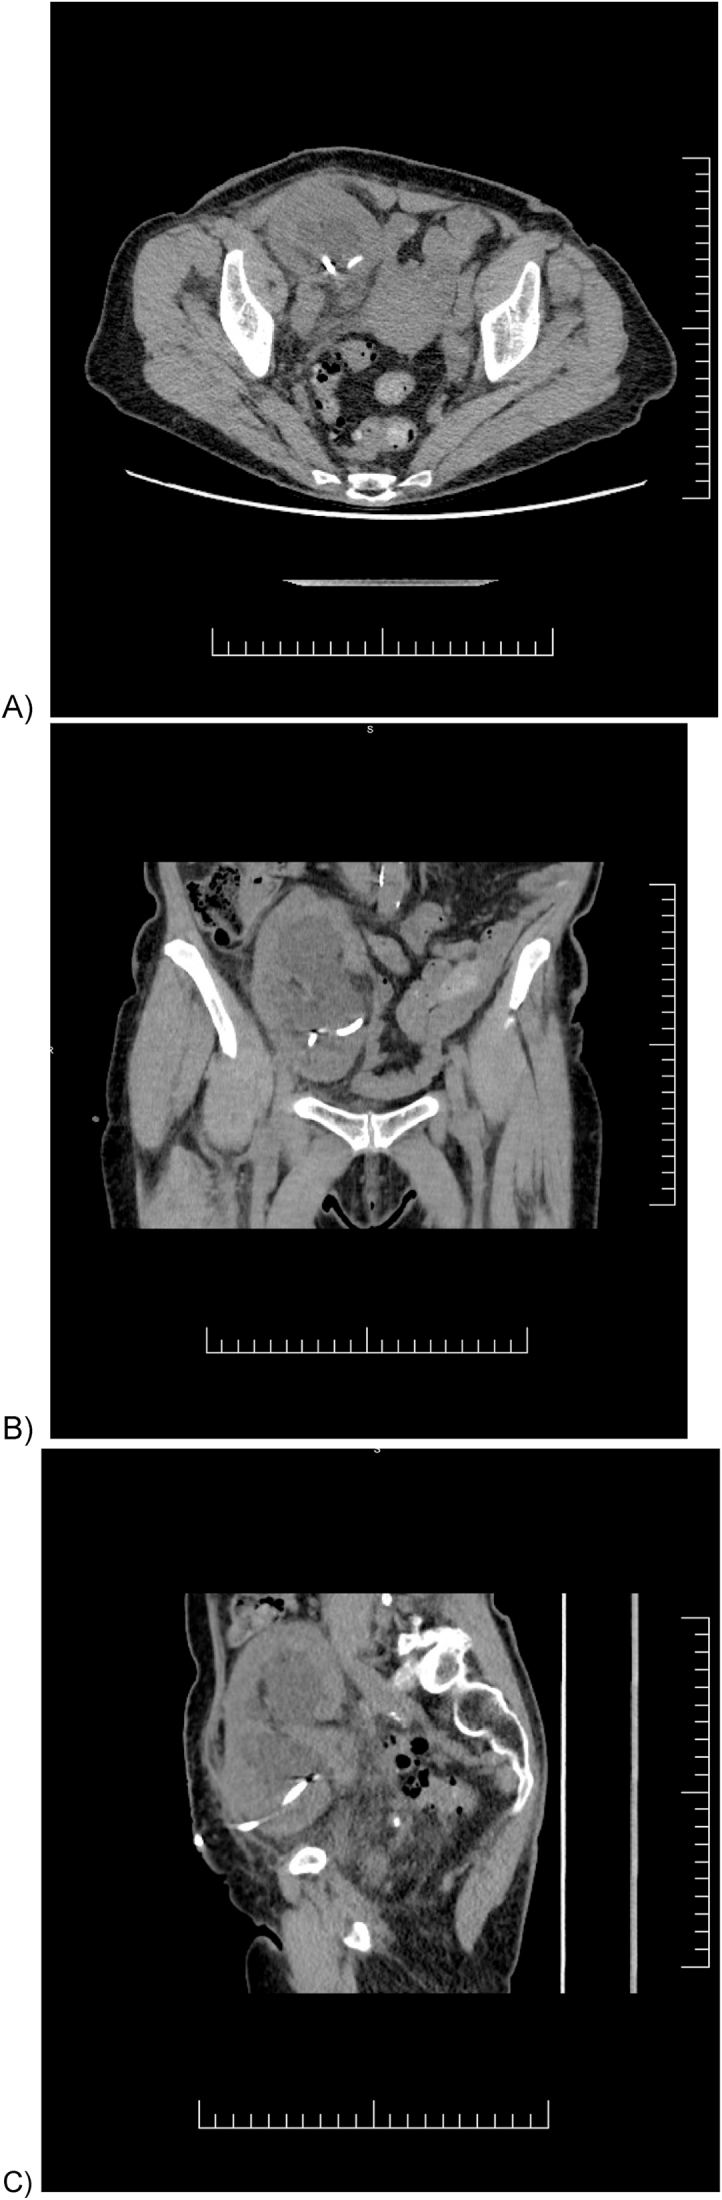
Non-contrast CT pelvis showing allograft kidney with possible graft hydronephrosis and nephrostomy tube (September 2024). A) axial, B) coronal, C) sagittal views.
